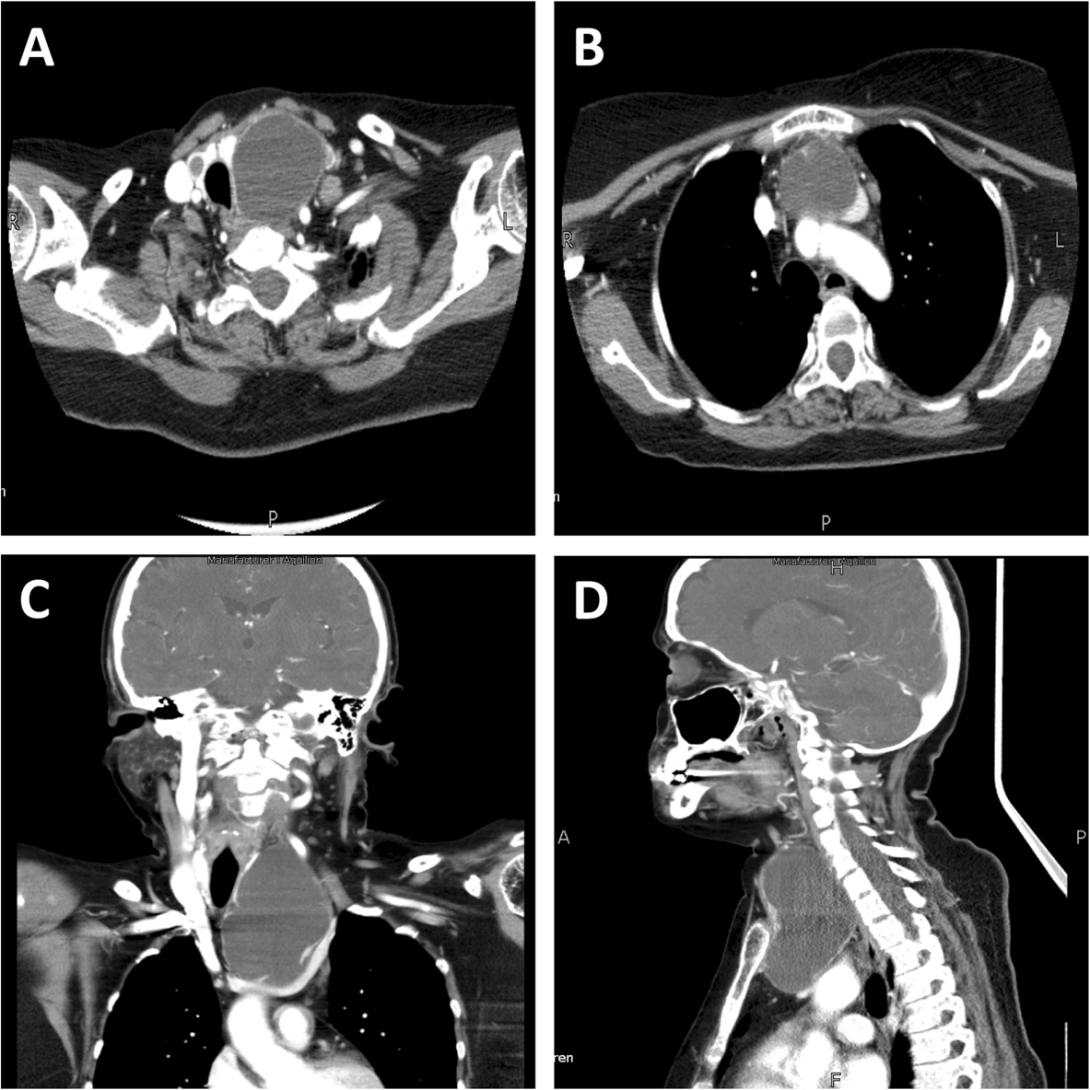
CT scan of head and neck showing parathyroid cystic mass extending into mediastinum.
